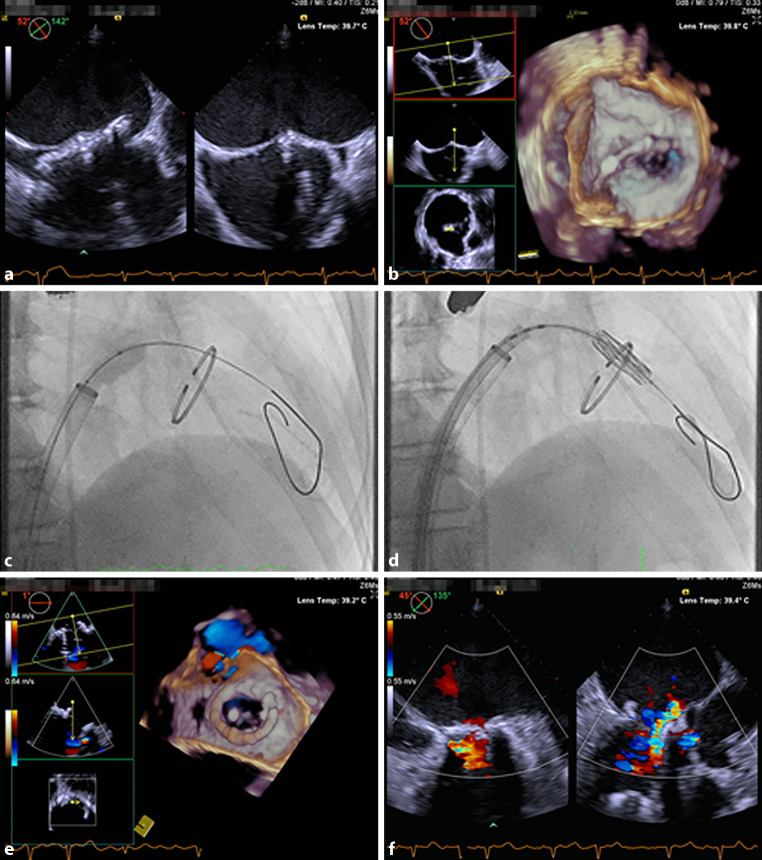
Transcatheter mitral valve replacement procedure. a, b Trans-septal puncture. c Trans-septal dilatation. d Balloon expandable transcatheter heart valve is deployed in mitral valve position. e, f Transoesophageal echocardiographic imaging confirms proper valve position and mild residual mitral regurgitation
